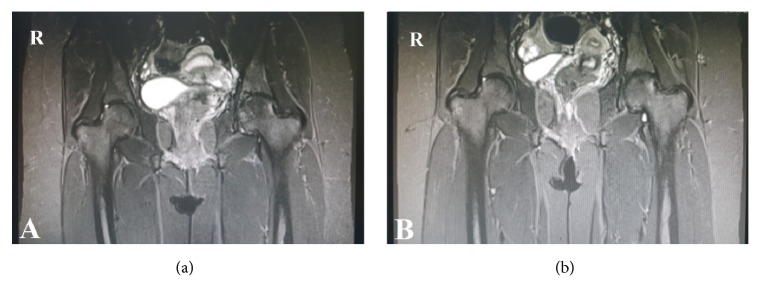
T1-weighted MRI image showing grade II ONFH of the left hip before ESWT (a). The lesion regressed to grade I at 2-year follow-up after ESWT (b).
